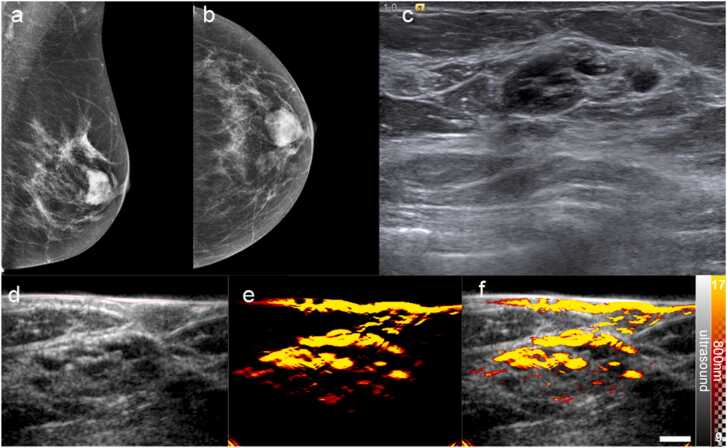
A false positive on OA-US. Mammography [MLO (a) and CC (b) views] reveals an oval mass in a retroareolar position. (c) Standard-of-care ultrasound reveals a complex cyst with multiple septations and a thick wall. (d) The US image from the OA-US scan at the same position is similar to the B Mode US. (d) The OA image from the OA-US scan at 800 nm reveals a pattern similar to that of disorganised vessels, which was interpreted as an irregular cap sign of malignancy, and the lesion was upgraded to malignant. (e) Overlaid OA-US image highlights the relationship between the area of high signal and the complex cyst. Scale bar 1 cm.
